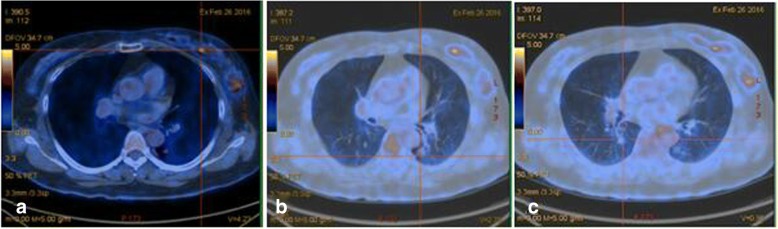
Positron Emission Tomography-computed tomography. The primary lesion in the left breast (a); The primary lesion in the left lower lobe of the lung (b) and the lesion in the right lower lobe of the lung (c)
